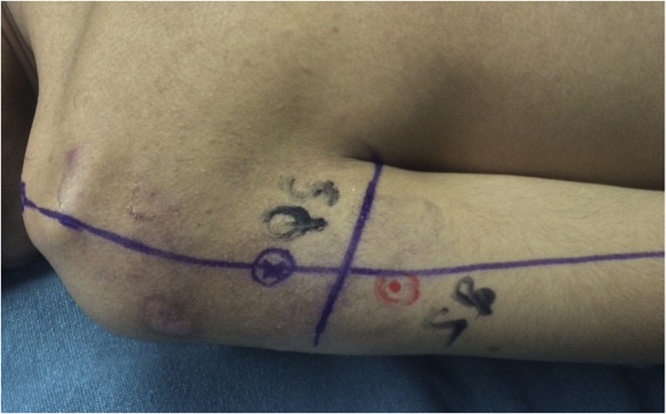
The red circle marks the localized cutaneous branch of the axillary nerve at the skin level (located by ultrasound).
